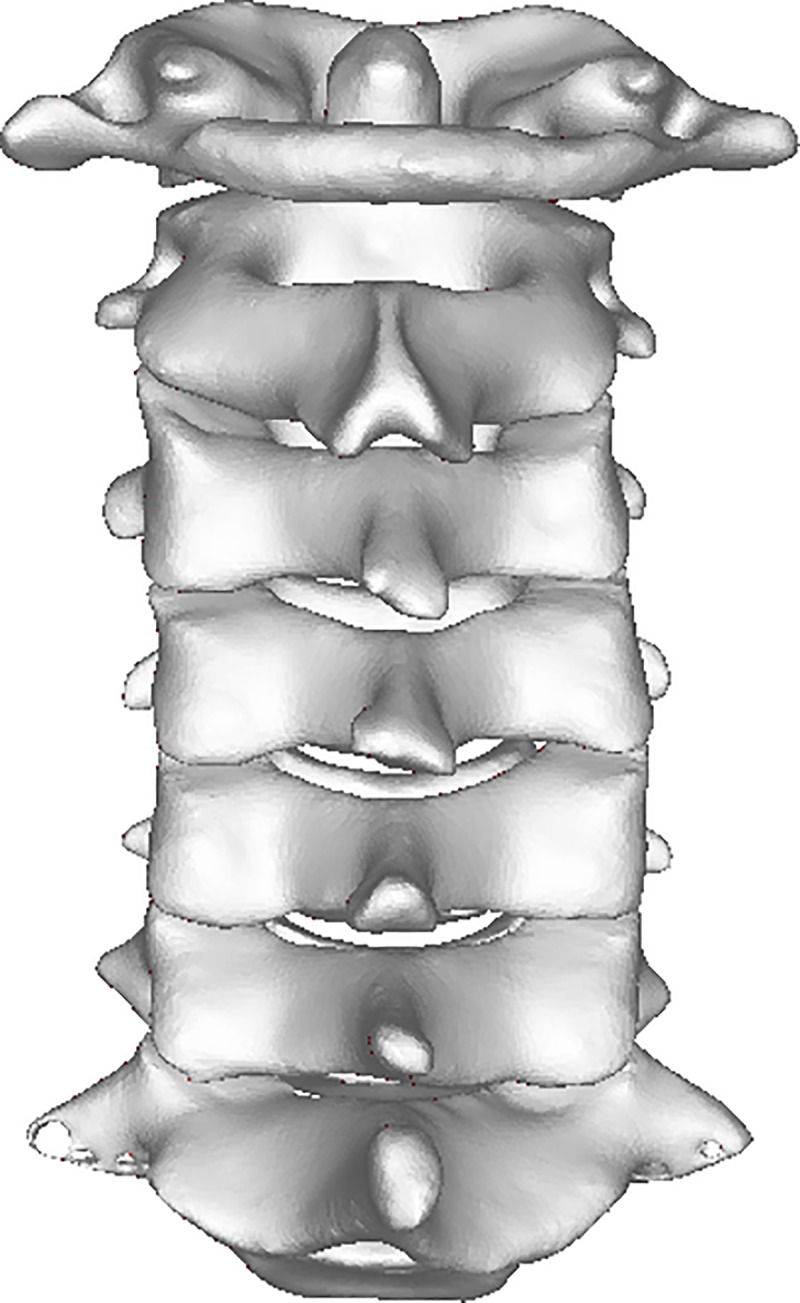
CT 3D reconstruction image of cervical spine: Posterior view.
